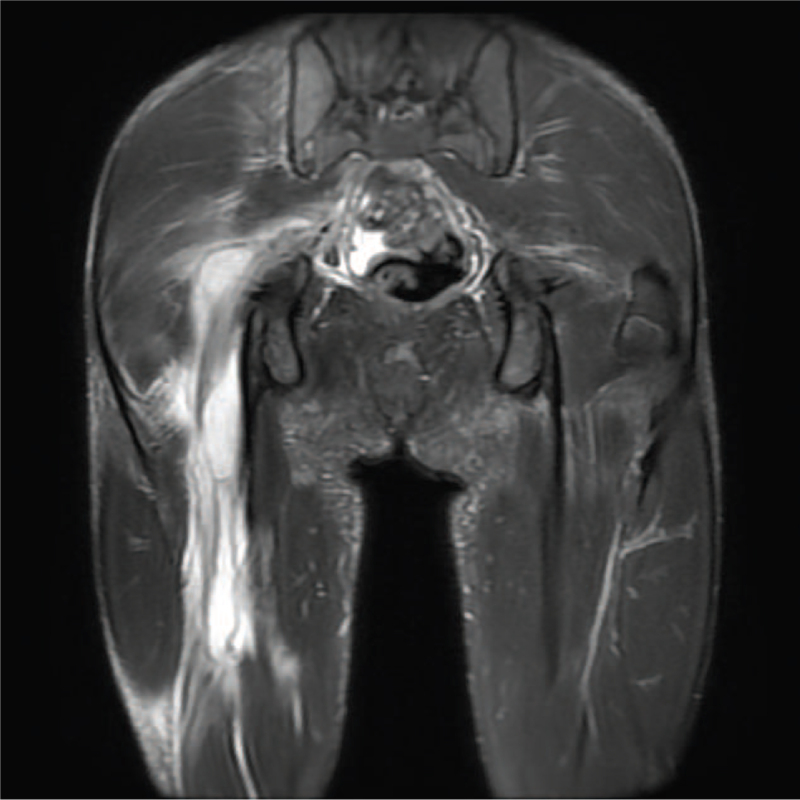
Magnetic resonance image (MRI) showing extensive abscess formation along right psoas muscle, right deep gluteal area, and right sciatic nerve.
